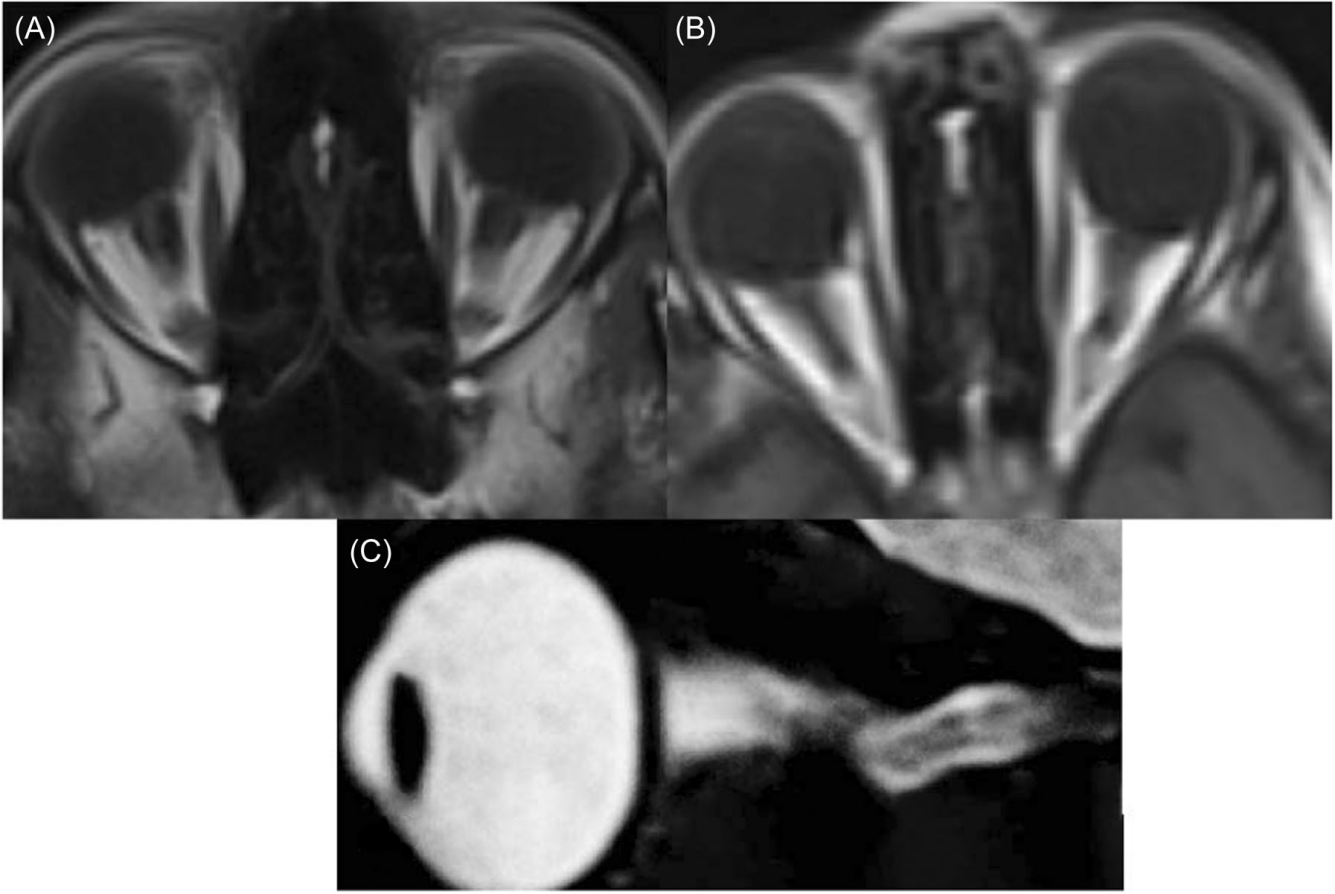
Optic nerve tortuosity. Loss of visualization of the middle portion of the orbital optic nerve and optic nerve tortuosity on T1‐weighted axial MRI is seen (A and B). An S‐shaped image of the optic nerve on sagittal images is seen (C). MRI, magnetic resonance imaging.
